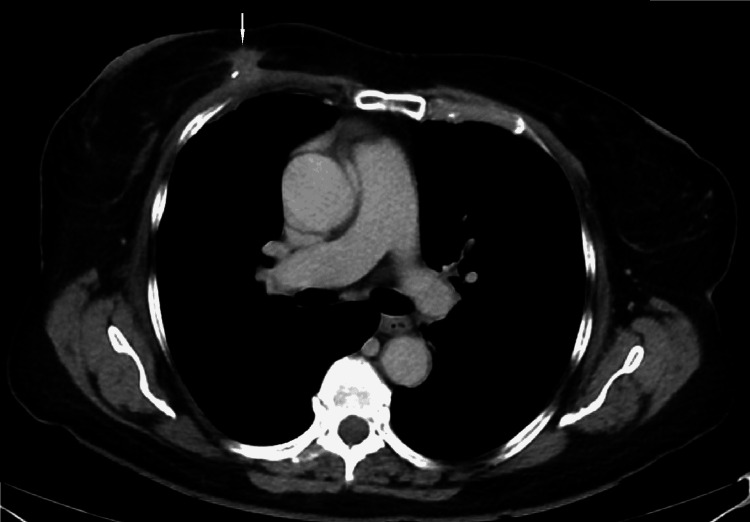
Contrast-enhanced CT at the time of presentation. Contrast-enhanced CT performed for staging demonstrates a mass in the central inner right breast at posterior depth inseparable from the right pectoralis musculature.
